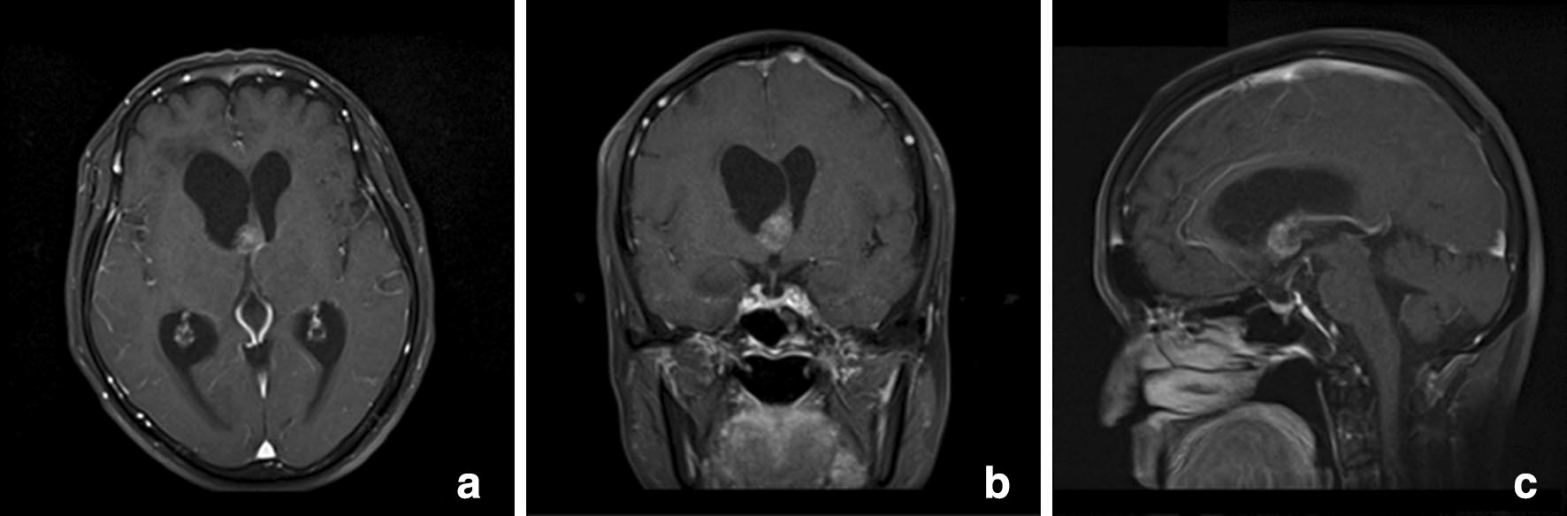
a Axial, b coronal, and c sagittal gadolinium-enhanced T1-weighted imaging demonstrated mild mass enhancement
